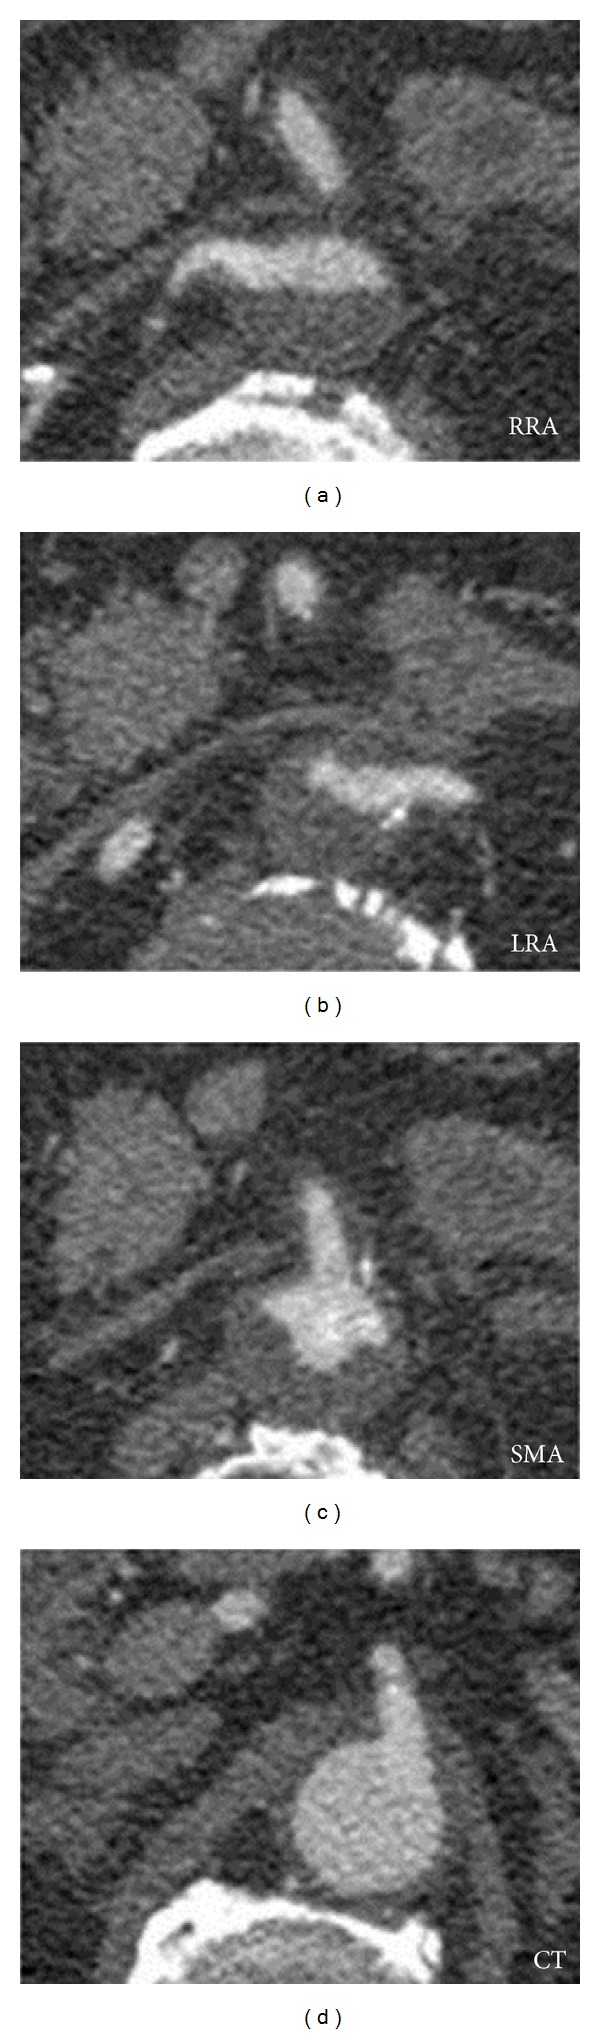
Axial view of the almost complete retrograde thrombosis to the level of the renal arteries and superior mesenteric artery (RRA: right renal artery; LRA: left renal artery; SMA: superior mesenteric artery; CT: celiac trunk).
